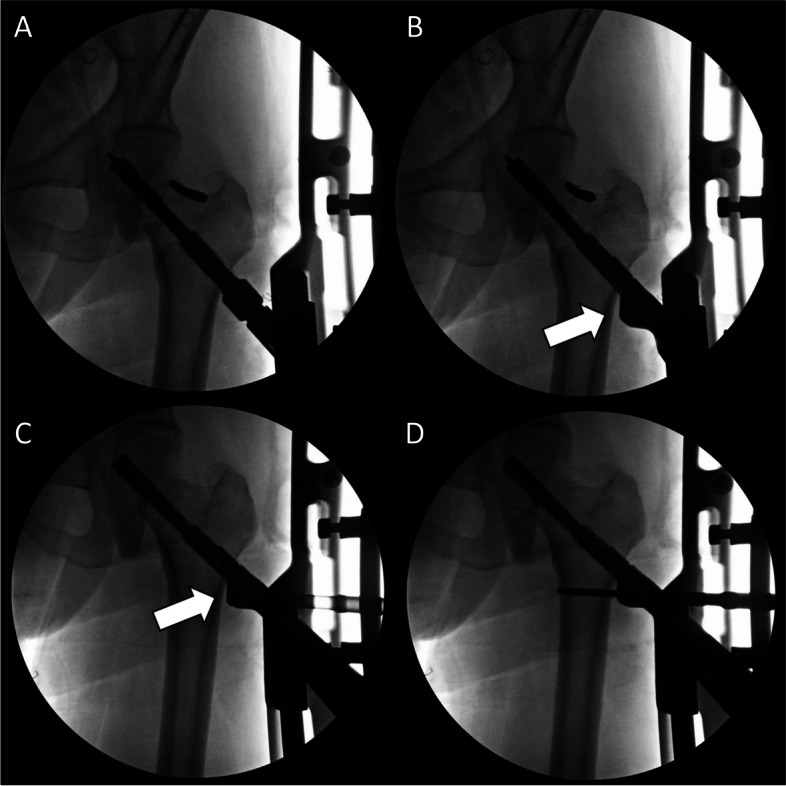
Indraoperative radiographs of the case shown in Fig. 7B show the surgical procedure for femoral neck fracture using the Femoral neck system. A After closed reduction using a Steinmann pin, a guide pin was inserted and reaming was performed. B The femoral neck system inserted with an insertion handle. According to our technique, the plate could not be contacted with the lateral femoral cortex (white arrow). C To reduce gap between plate and lateral femoral cortex (white arrow), the black screw was loosened and the plate was impacted. D After then, locking screw was inserted. The photographs were taken by the authors
